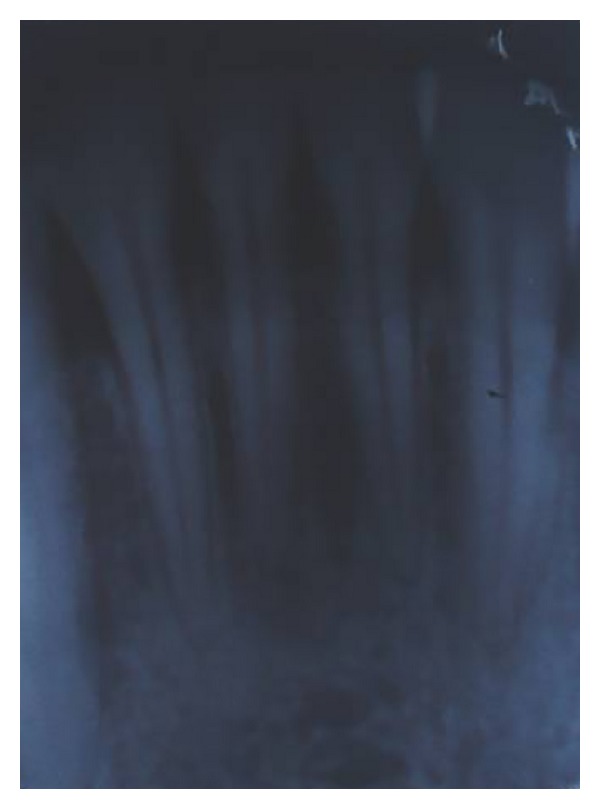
Radiograph in relation to lower anterior region showing absence of interdental bone loss.
